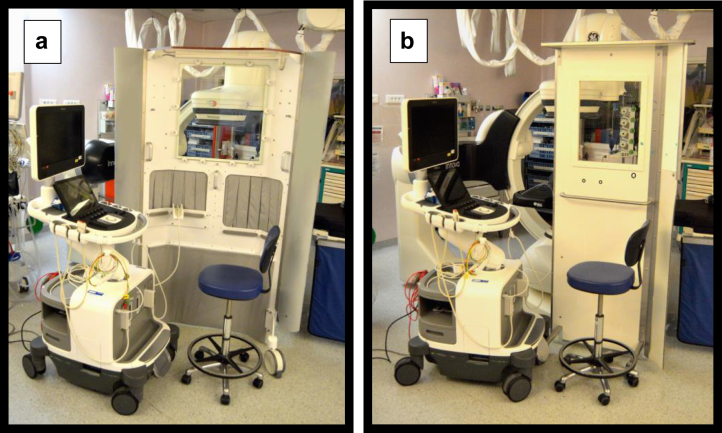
Photos of (a) the Echosafe cabin and (b) the control radiation protection device. (a) The Echosafe cabin allows introducing the transoesophageal probe through the left lead flap and the arm of the echocardiographer through the right flap. The X-ray tube is close to the patient’s head on the other side of the cabin, and the interventional cardiologists are located on the right of the cabin (radial and femoral approach). (b) The control radiation protection device, which was used previously in our hospital, is a lead glass door placed between the echocardiographer and the X-ray tube.
